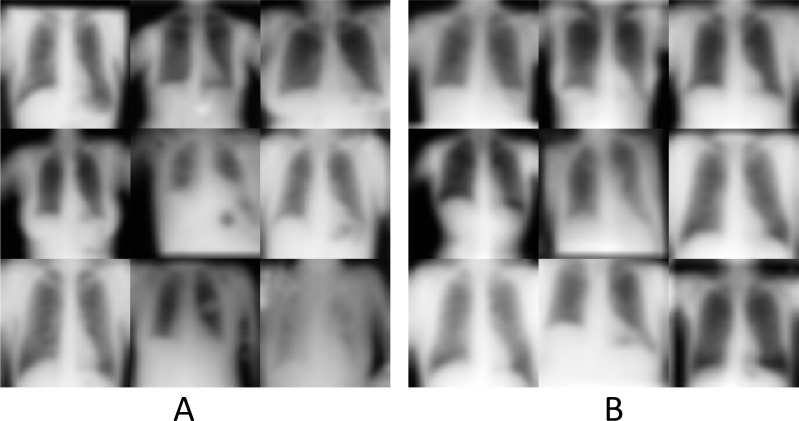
(A), Examples of the original images from the ChestMNIST subset in MedMNIST v2; (B), Examples of chest X-ray images generated using Huber loss after 40 epochs of training.
